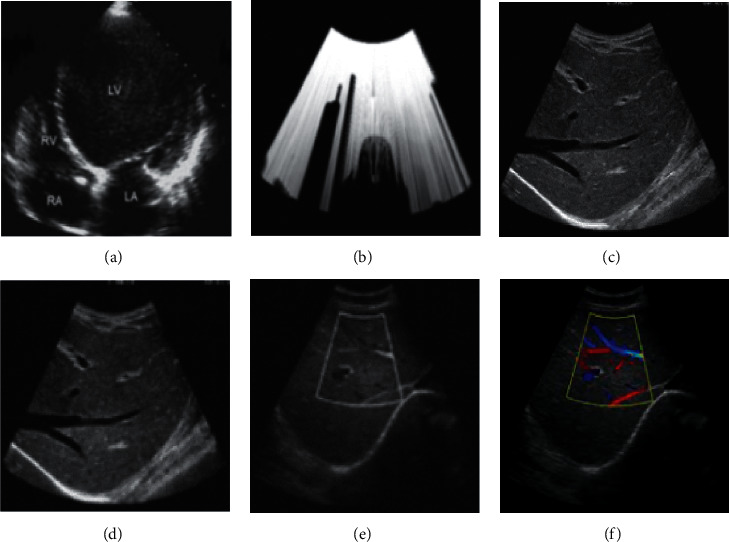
Ultrasound image and propagation attenuation image.
